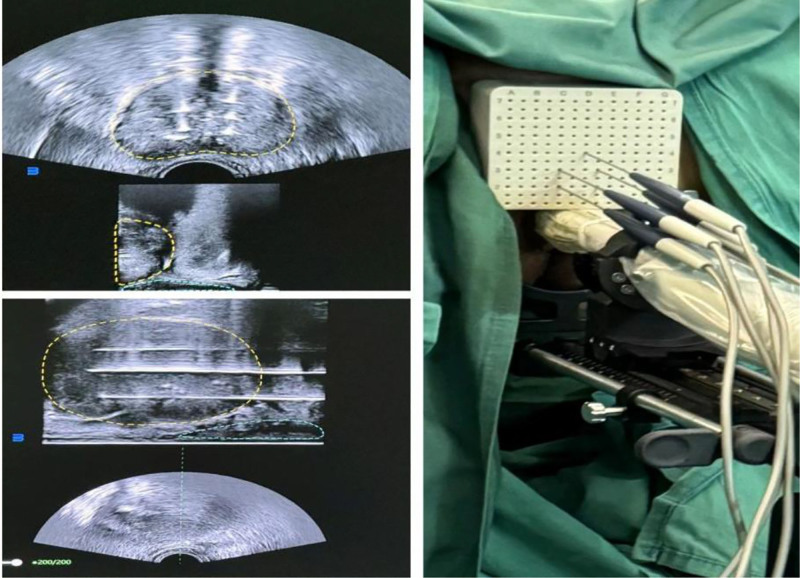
Intraoperative ultrasonic positioning operation.
